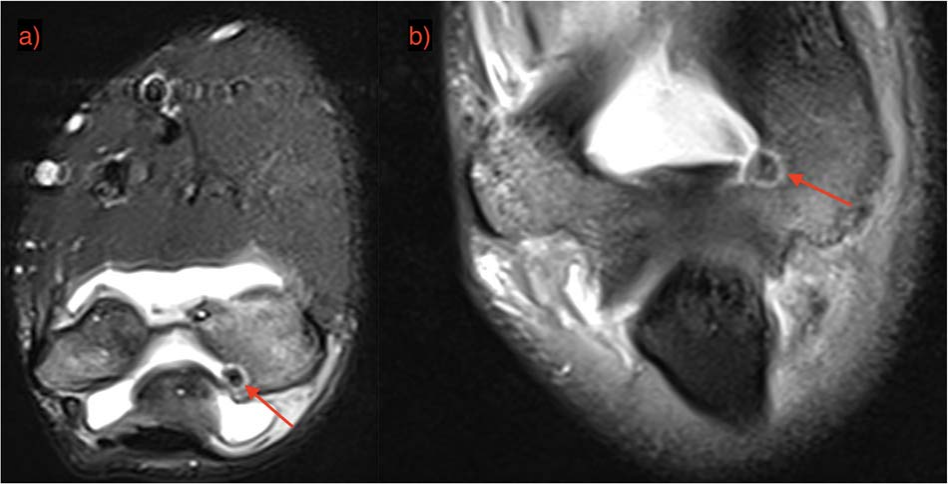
OO localization with contrast MRI: (a) coronal view and (b) frontal view.
